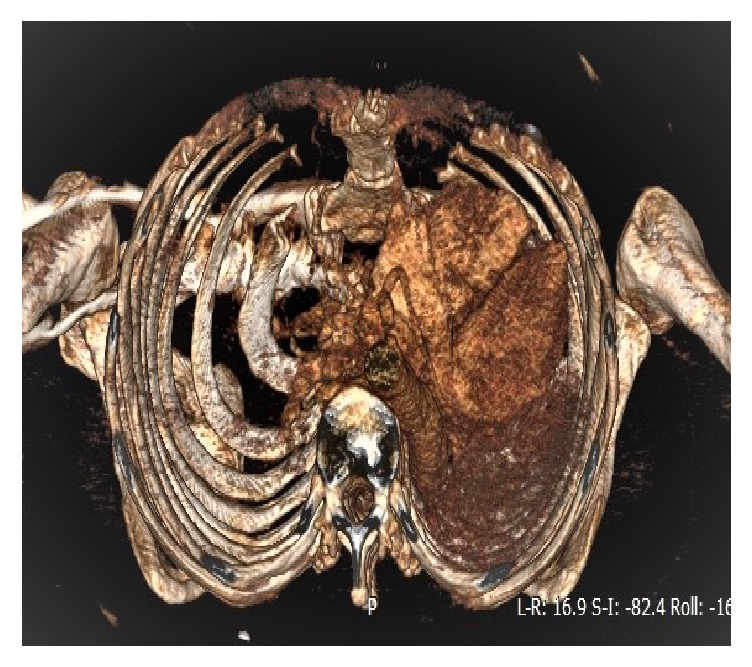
3D CT scan in axial tomography of the chest confirmed a total left lung atelectasis.
